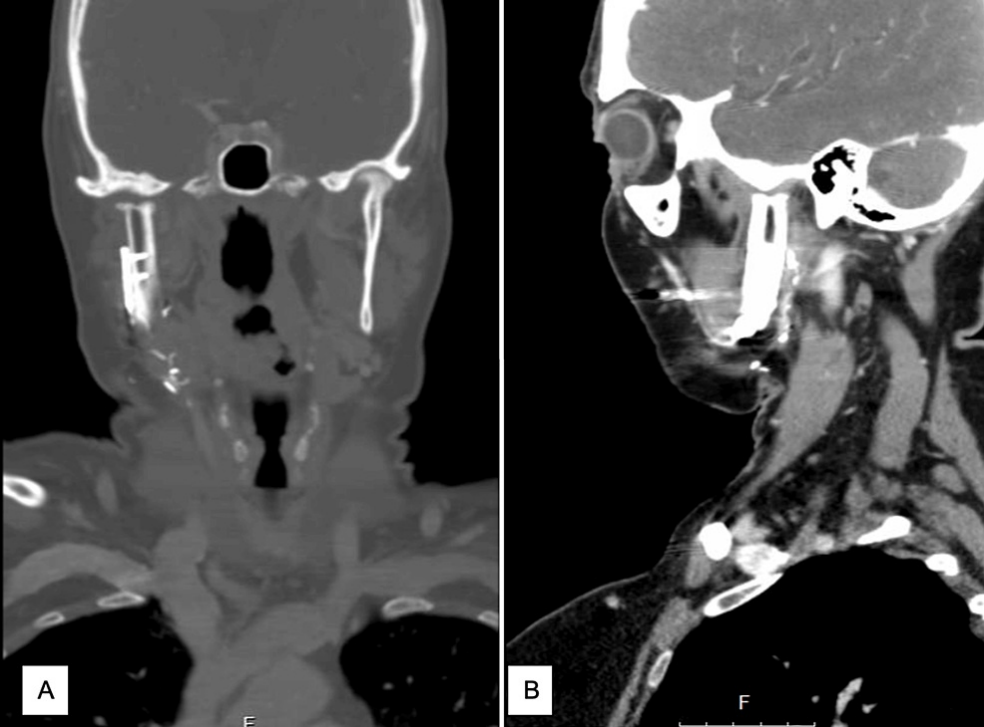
CT imaging of neo-condyle within the glenoid fossa in coronal view (A) and sagittal view (B).
